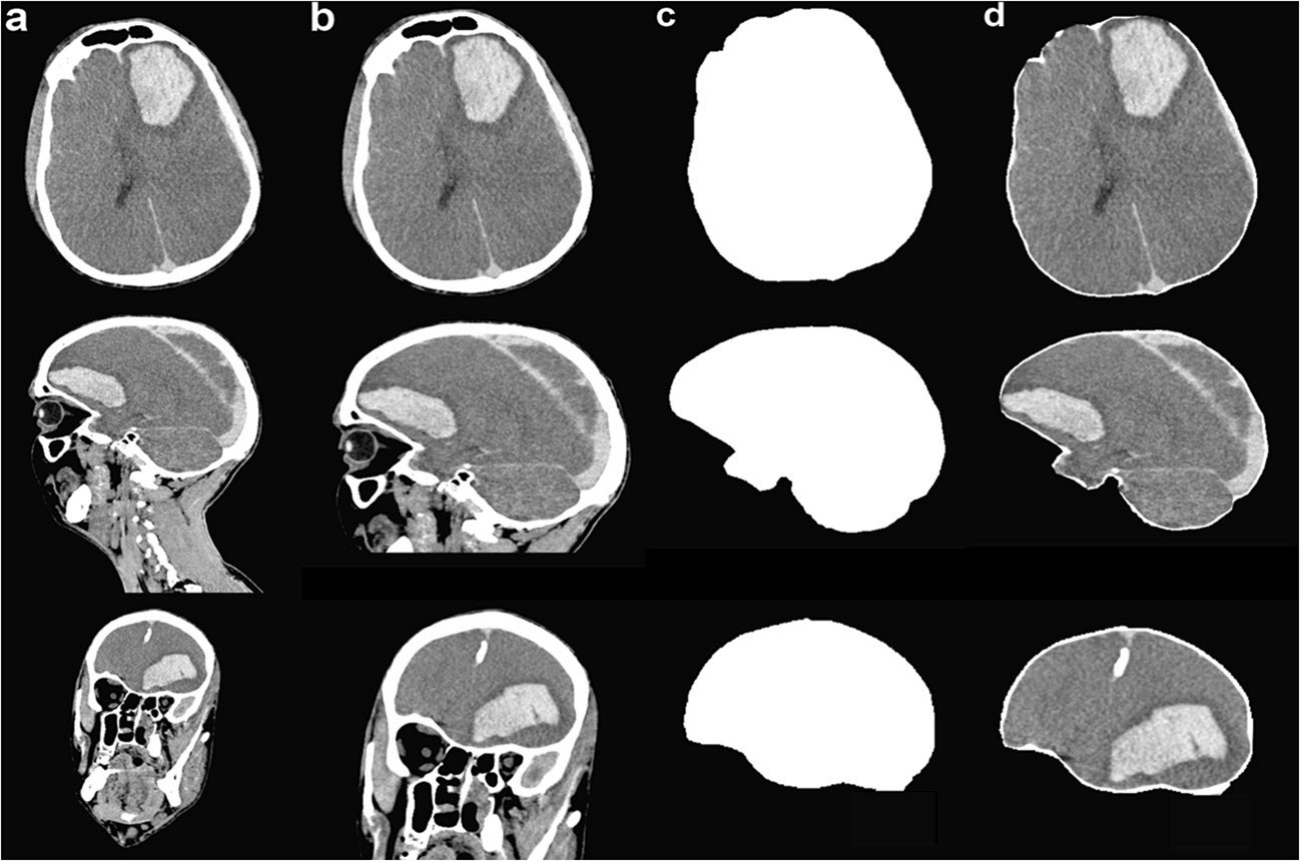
Exemplary visualization of the image segmentation process. Top: axial plane, middle: sagittal plane, bottom: coronal plane. a Original CT images (windowed), b cropped images with size 420 × 420 × 420 px, c mask created by FSL, and d segmented brain images
